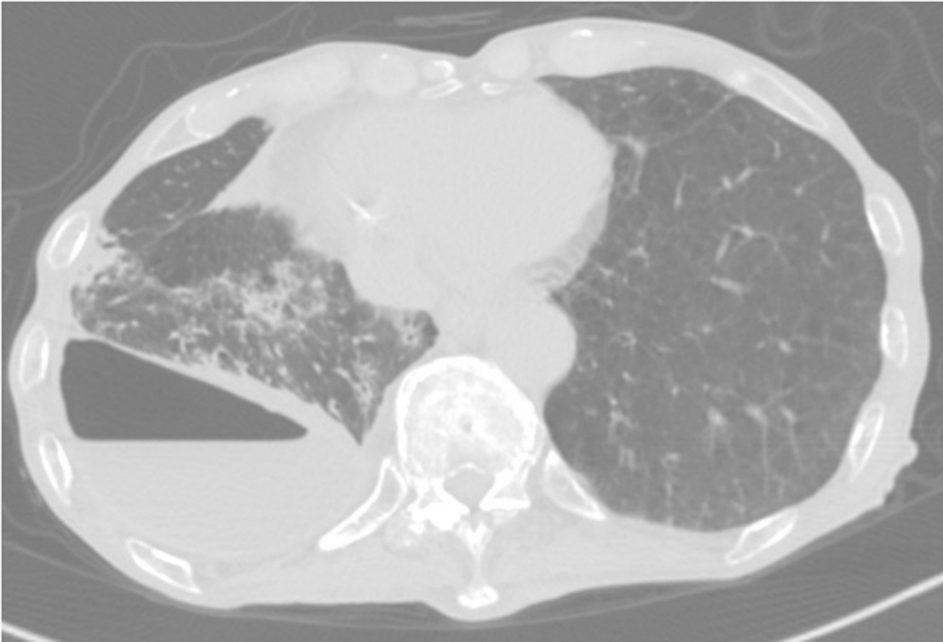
CT scan showing pneumothorax and centrilobular nodular shadows with ipsilateral increasing pleural effusion.
